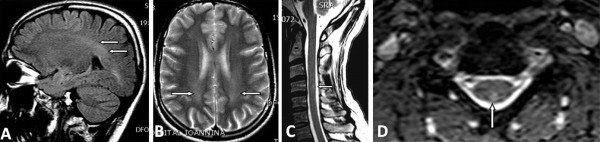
Magnetic resonance imaging (MRI) lesions compatible with demyelinating disease. Sagittal fluid attenuated inversion recovery (A) and axial T2-weighted (B) scans show bilateral diffuse hyperintense signal in the periventricular white matter of the parietal and occipital lobes (arrows). T2-weighted sagittal (C) and axial (D) spinal cord scans reveal a small peripherally located lesion (arrows).
